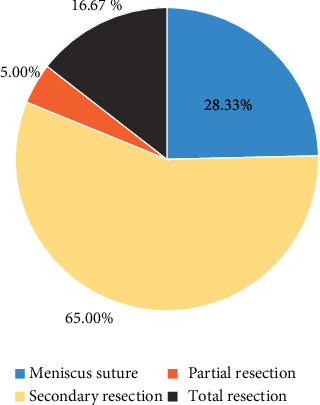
Statistics of treatment methods of MRI-diagnosed meniscus injury.
